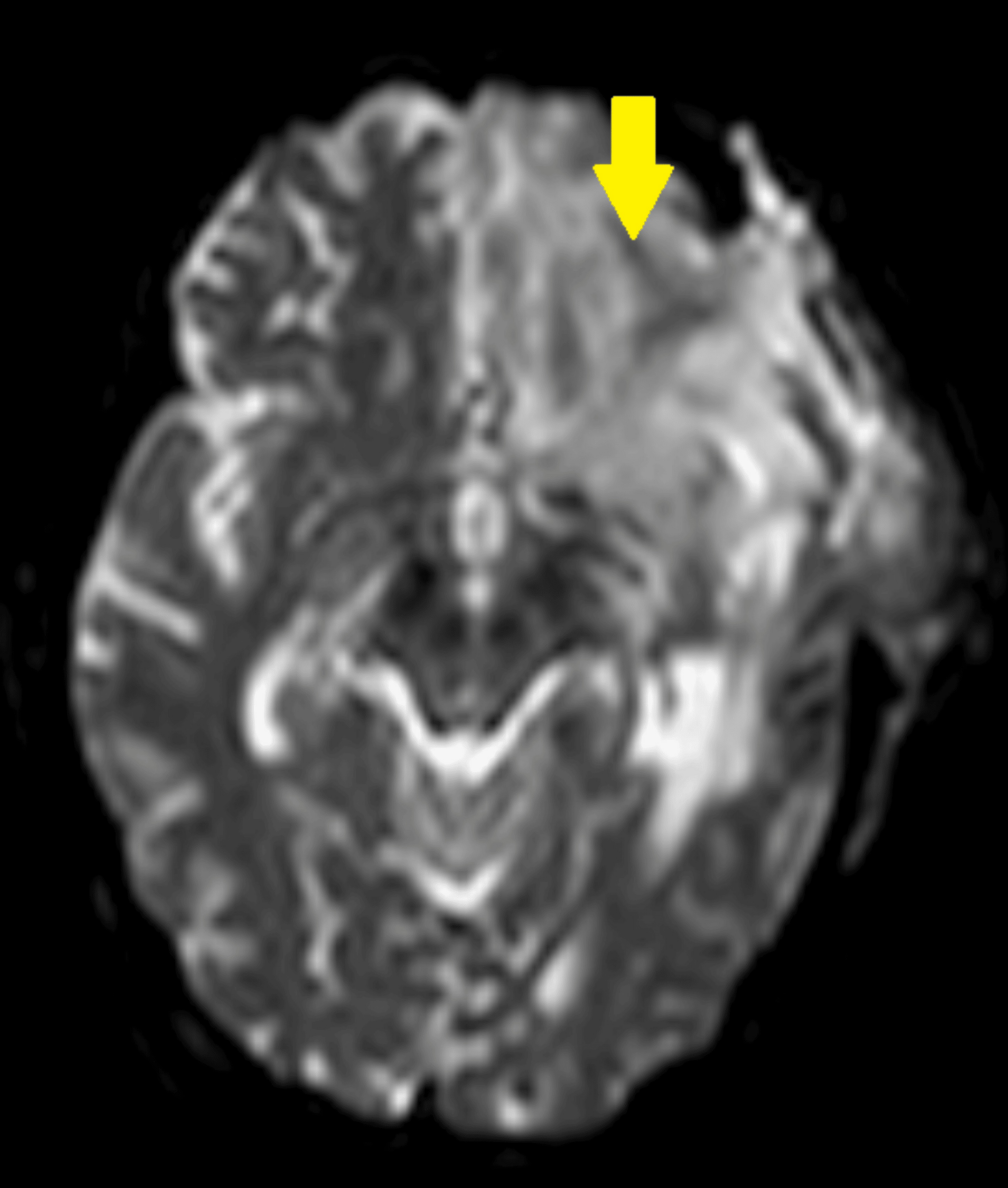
Axial DWI MRI Brain Without Contrast Image quality is limited by patient movement, and the study ended early after the patient was unable to tolerate lying supine for the entire duration of image capture. Although image interpretation is limited by the resolution, it demonstrates evolving late subacute infarction in the left anterior and middle cerebral artery distributions (yellow arrow). DWI: diffusion-weighted imaging.
